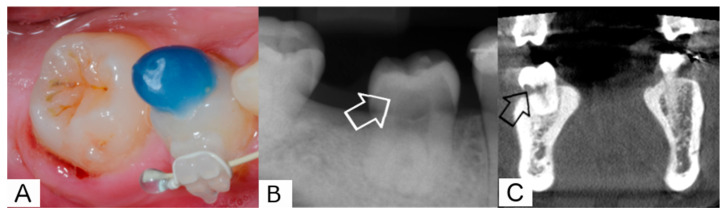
18-month follow-up. (A). Clinical picture with signs of inflammation (bleeding and suppuration from the gingival margin) (B). Intraoral radiograph with suspect radiolucency in the crown of the transplant (white arrow) (C). Native CBCT (resolution 0.2 mm) The arrow shows the buccal resorption in the subgingival part of the crown of the transplanted tooth, but otherwise a regular periodontal ligament space and no further signs of external root resorption.
